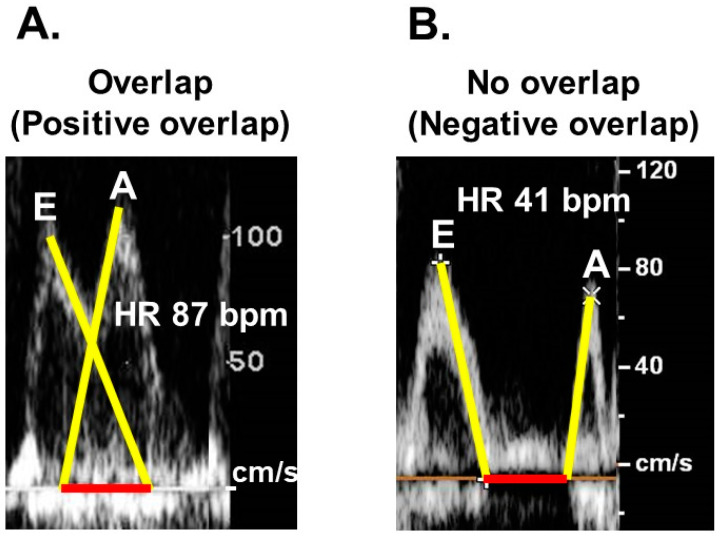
Trans-mitral Doppler echocardiography in patients with an obvious overlap (positive overlap) between the E-wave and A-wave due to a relatively higher heart rate (A) and in patients with no overlap (negative overlap) between the two waves due to a relatively lower heart rate (B) (with permission to reuse from the publisher) [11]. The obvious wave-overlap indicates insufficient left-ventricular filling time and results in lower cardiac output. The obvious wave-separation indicates sufficient left-ventricular filling time but results also in lower cardiac output due to lower stroke number.
